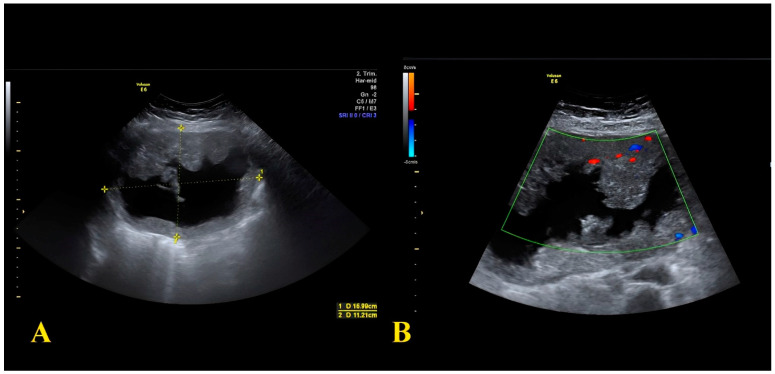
(A) Sonographic image showing the 16 × 11 cm adnexal cyst with a solid component; (B) Sonographic image showing intralesional vascularization.
